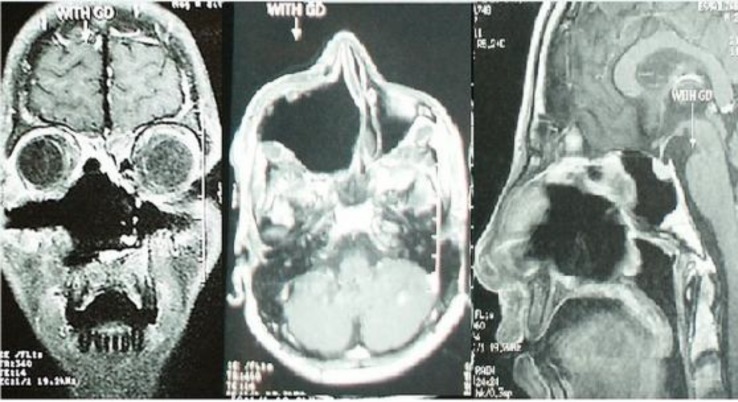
Postoperative MRI, Coronal, axial and sagittal T1 with Gd-DTPA which showed complete removal of the tumor
